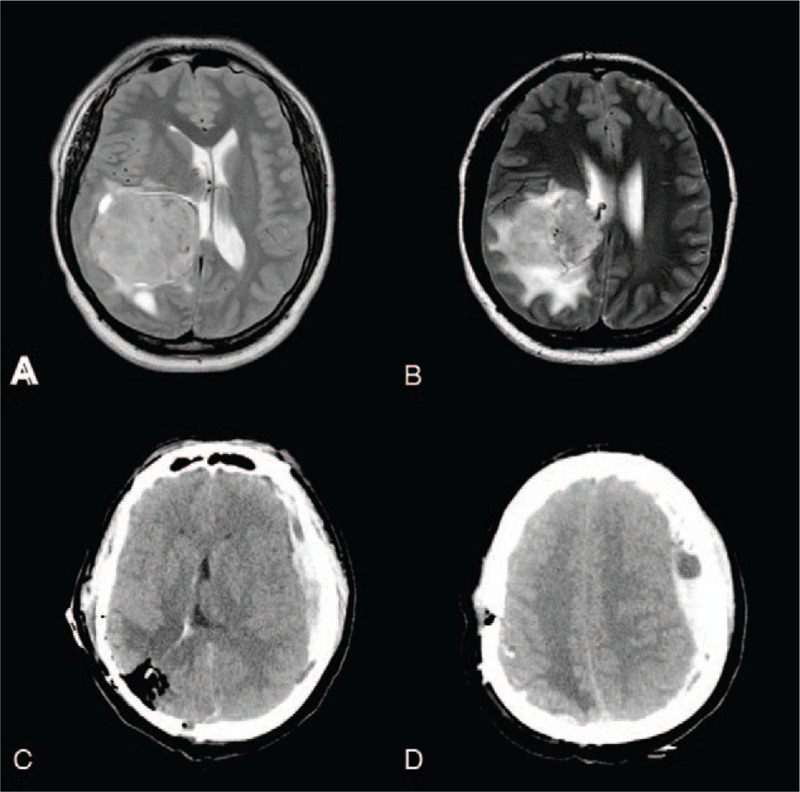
Radiological profiles of Case 1. (A–B) Axial T2-weighted MRI revealed a lesion in the right posterior horn of the lateral ventricle, with remarkable peritumoral edema. (C–D) Postoperative CT revealed a contralateral subdural hematoma. CT = computed tomography, MRI = magnetic resource imaging.
